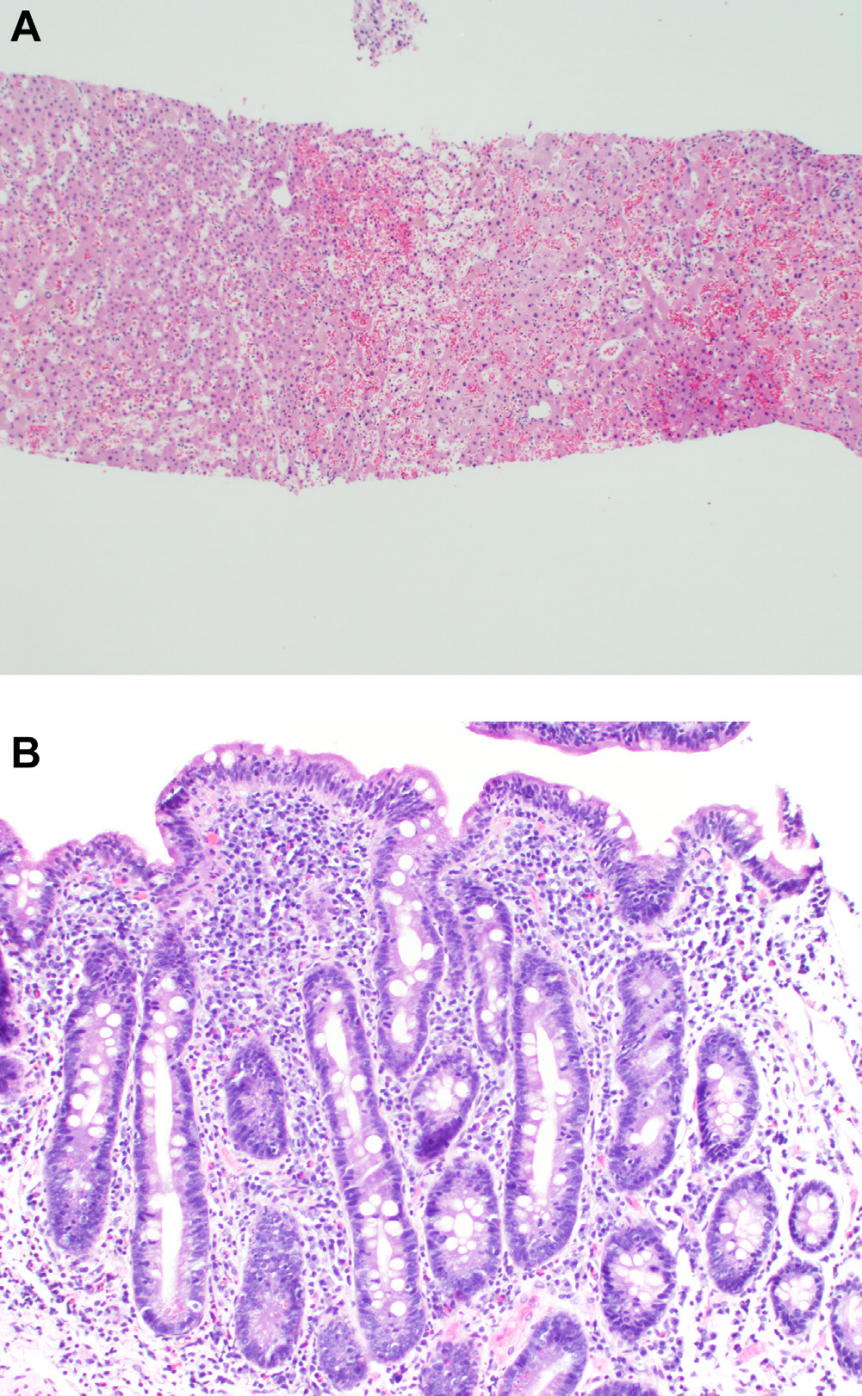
When seen in consultation, pertinent observations included Irish ancestry, a history of occasional diarrhea, short stature, osteoporosis, and an atrophic spleen on computed tomography. An Immunoglobulin A transglutaminase antibody was positive, and a small bowel biopsy confirmed celiac disease (Figure B). On a gluten-free diet, her symptoms resolved, with clinical and laboratory abnormalities returning to normal. She lived another 20 years before dying of primary pulmonary hypertension. Recognition of an unusual hepatic manifestation of celiac disease led to effective management.
